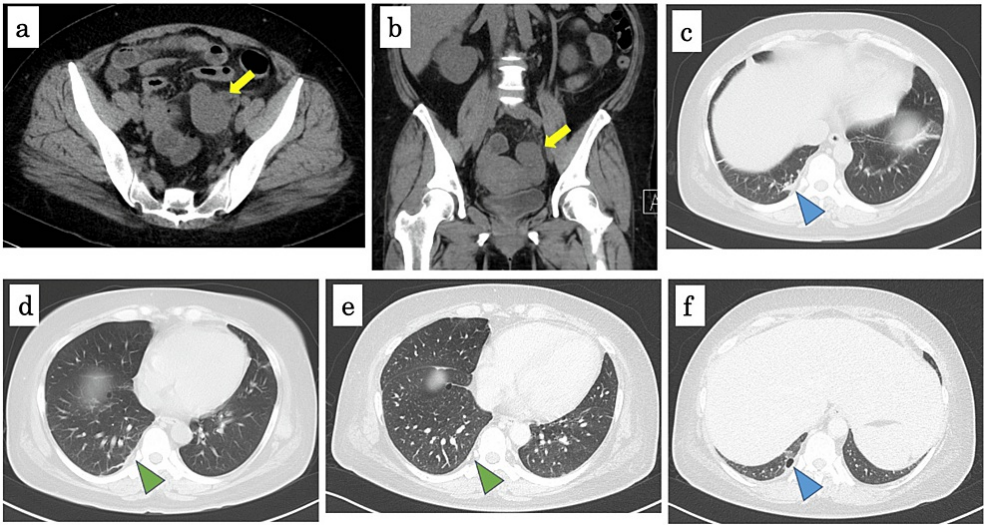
CT on admission (a-d) and on day 5 after admission (e-f) (a, b) Abdominal CT on admission demonstrated that the left side of uterine appendages was enlarged (yellow arrows); (c, d) Chest CT on admission demonstrated that small consolidation was located beside the pleura of the lower lobe of the right lung (blue arrow) and the pleura was thickened mildly (green arrow); (e, f) Chest CT on day 5 after admission demonstrated that the pleura was not thickened (green arrow) and the consolidation turned to bulla (blue arrow).
